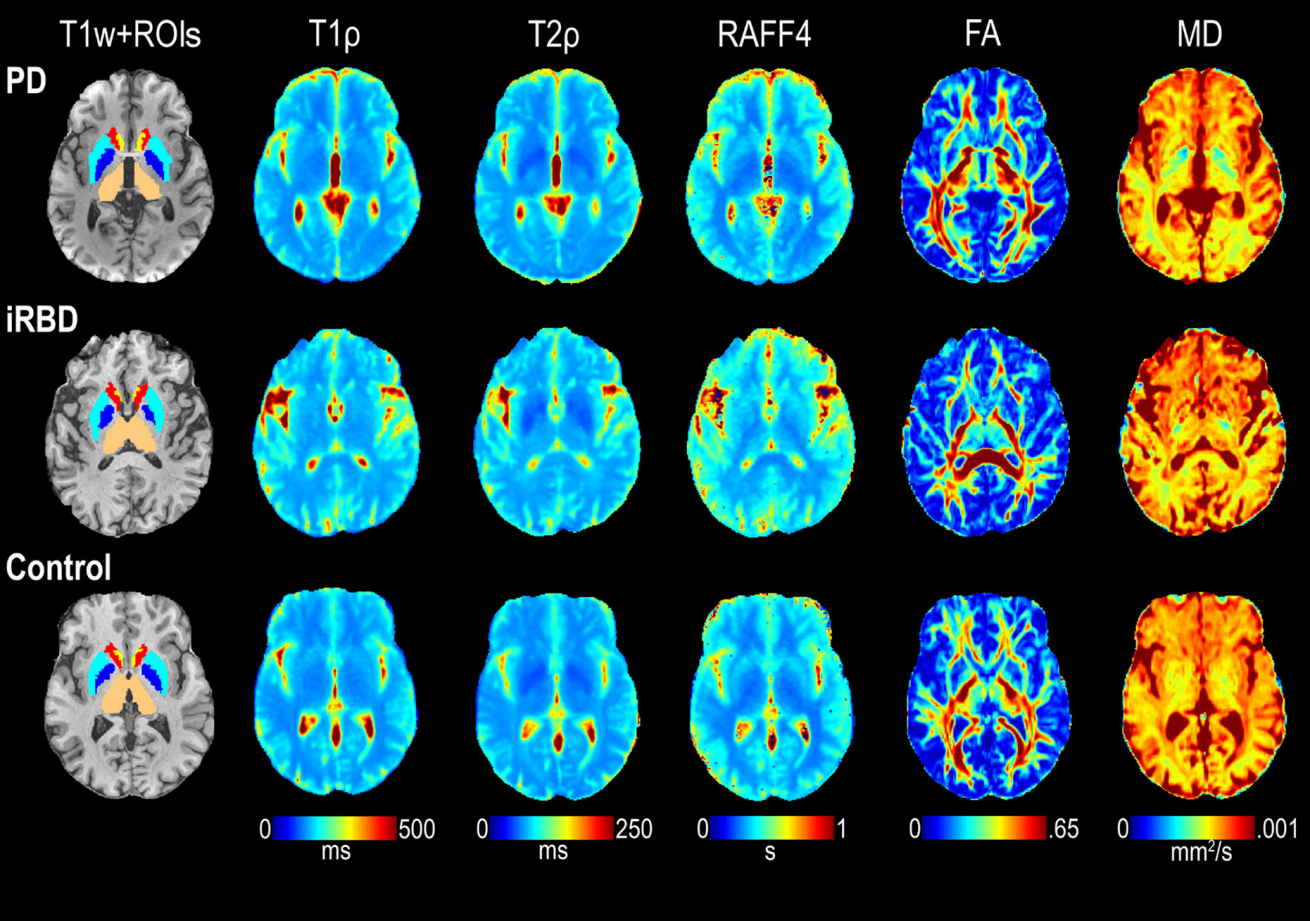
Representative maps of T_1ρ_, T_2ρ_, RAFF4, FA, and MD from one each of a control, iRBD and PD subject. ROIs of interest visible on the selected slices are also shown superimposed on the T1-weighted (T1w) images, including thalamus (beige), pallidum (dark blue), putamen (light blue), caudate (red), and accumbens (yellow).
